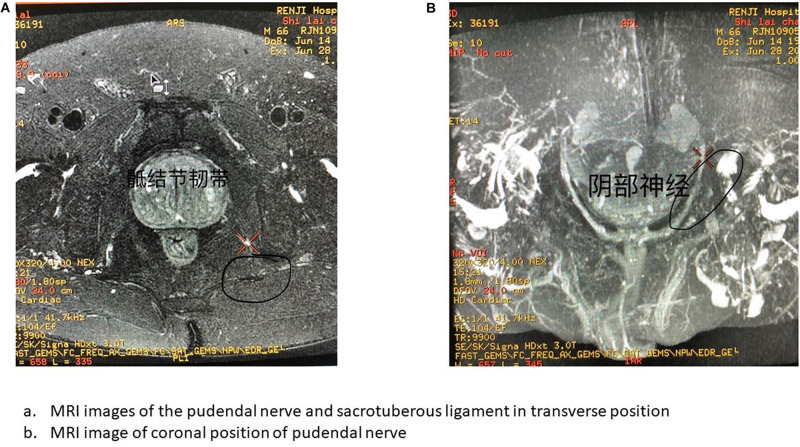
MRI image of pudendal nerve. (A) MRI images of the pudendal nerve and sacrotuberous ligament in transverse position. (B) MRI image of coronal position of pudendal nerve.
